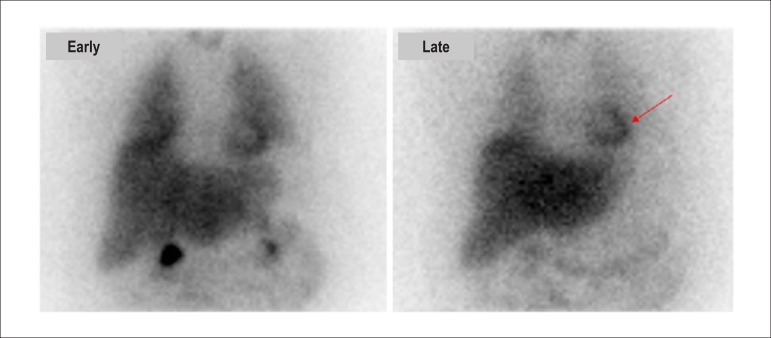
Cardiac scintigraphy with MIBG-^123^I showing normal radiopharmaceutical uptake in the cardiac area (arrow), which denotes preserved cardiac sympathetic activity. Black and white images of the anterior thorax, acquired approximately 15 minutes (early) and 4 hours (late) following intravenous injection of the radiopharmaceutical MIBG-^123^I.
